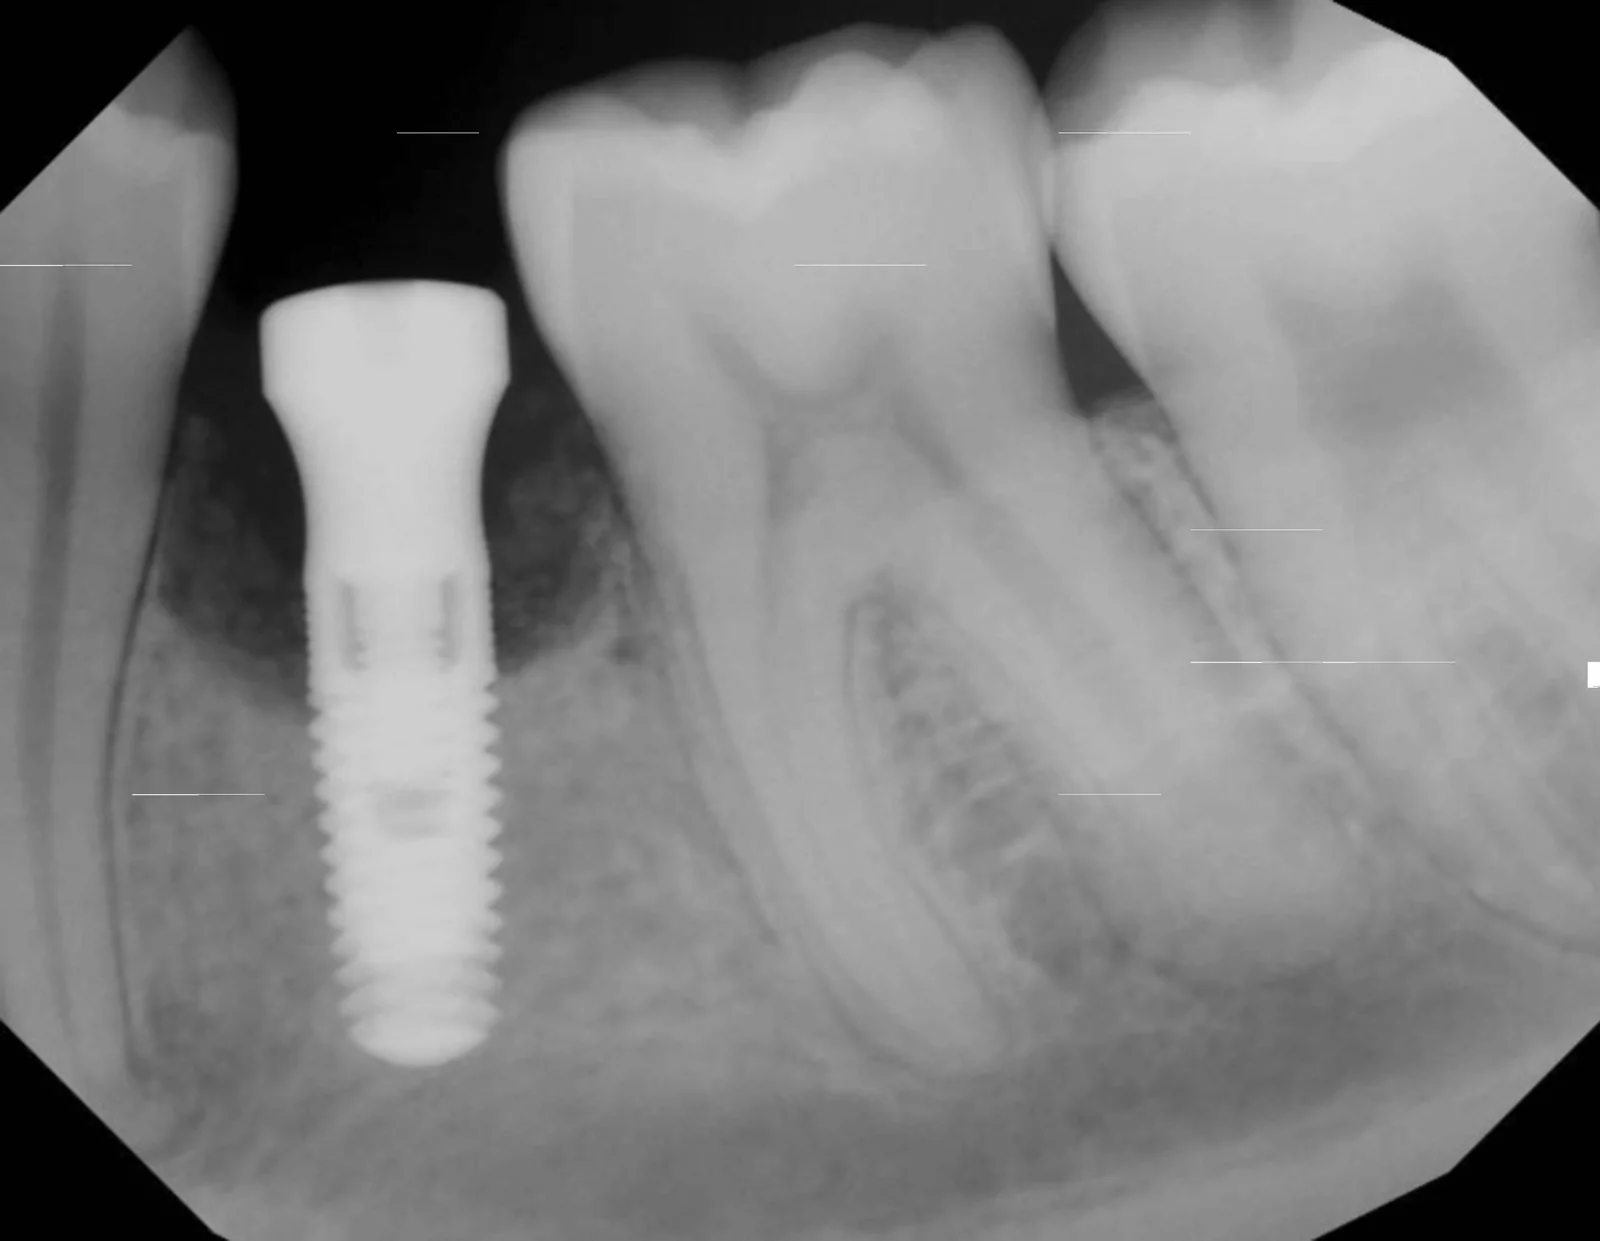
Conventional periapical radiograph of the implant site. The two-dimensional image provides an overall view of the implant and adjacent bone but may obscure facial or lingual peri-implant changes.
